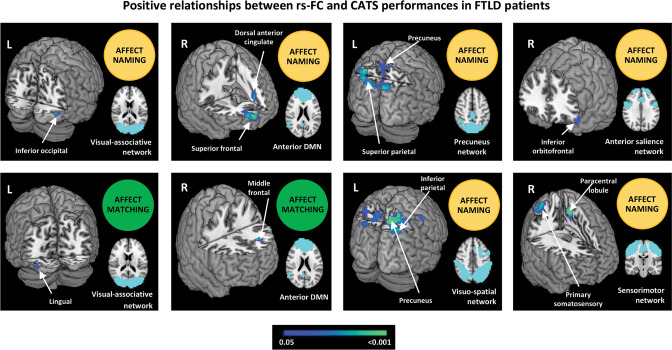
Independent component analysis. Positive relationship between resting state functional connectivity and CATS scores in FTLD patients. Positive relationships are shown in cold colors. Results are overlaid on the Montreal Neurological Institute (MNI) standard brain and displayed at *p* < 0.05 Family-wise error corrected for multiple comparisons. Age at MRI, sex and education were included in the model as nuisance variables. rs-FC resting state functional connectivity, L Left. Color bar represents *p* values.
